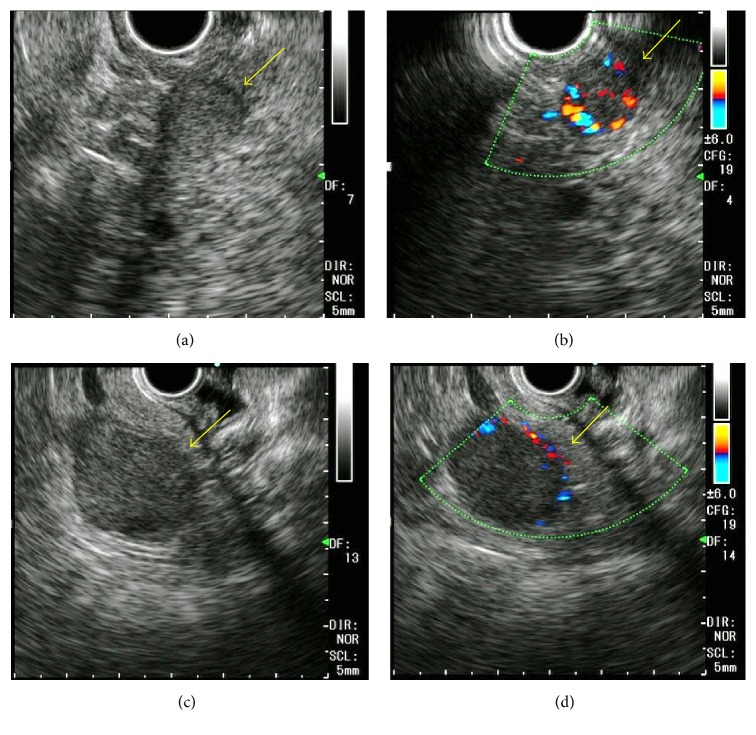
Endoscopic ultrasonography (EUS) imaging sections of pancreas tumors*. Pancreatic tumors are shown by Convex EUS. A tumor in the head of the pancreas is shown as yellow arrows in (a) and (b) and that in the tail of the pancreas is shown as yellow arrows in (c) and (d). Both tumors had similar findings and hypervascularity in tumor was demonstrated by Doppler mode of EUS.
